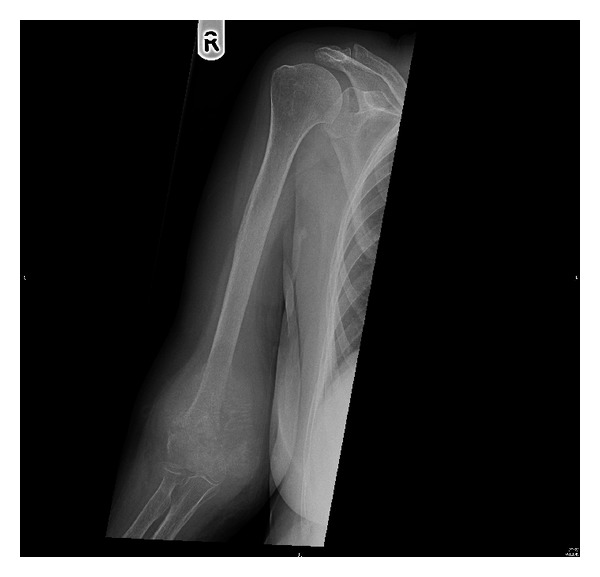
Plain radiograph of right arm showing aggressive bony lesion involving the distal metadiaphysis and epiphysis of the right humerus and associated with the sunburst periosteal reaction and pathological fracture.
